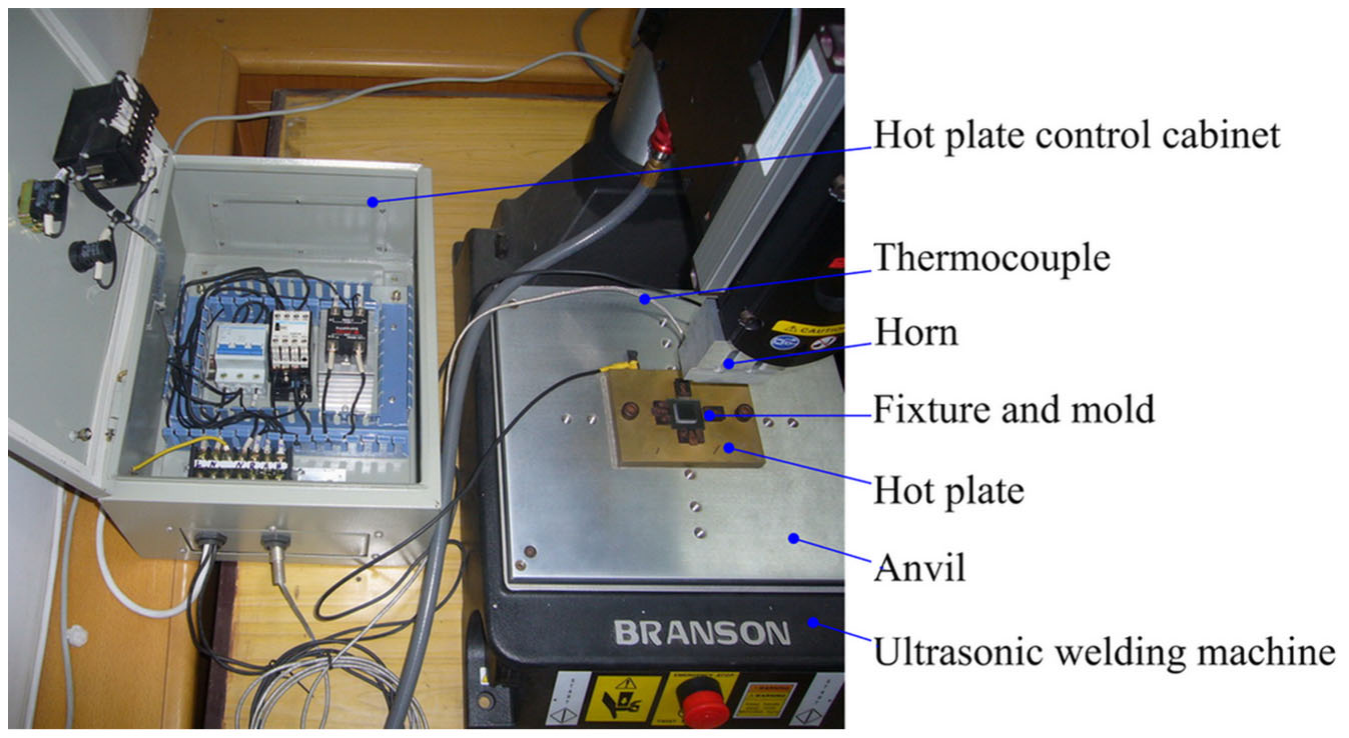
Thermal assisted ultrasonic embossing system.
